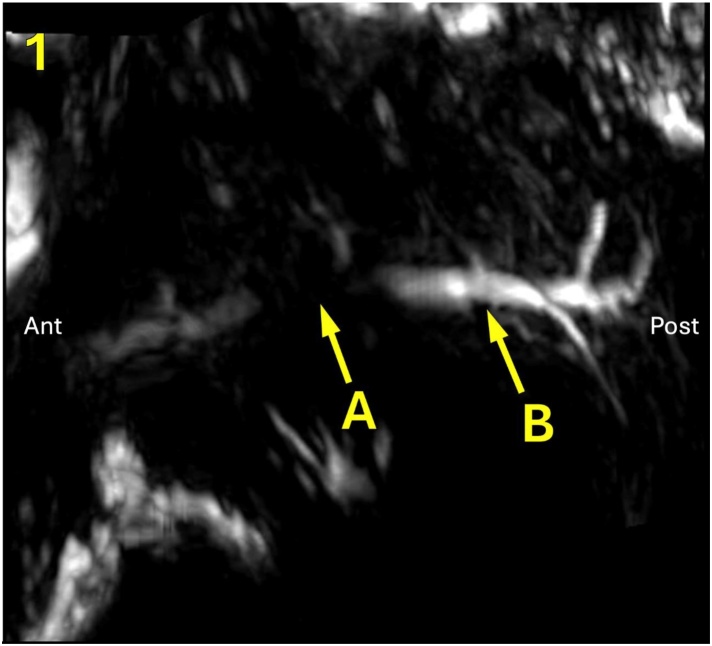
Magnetic resonance imaging showing fillers causing parotid duct stenosis: MRI profile, sagittal section with parotid duct stenosis due to compression by filler (Silicone). (A) Duct filling failure. (B) Proximal duct dilation.
